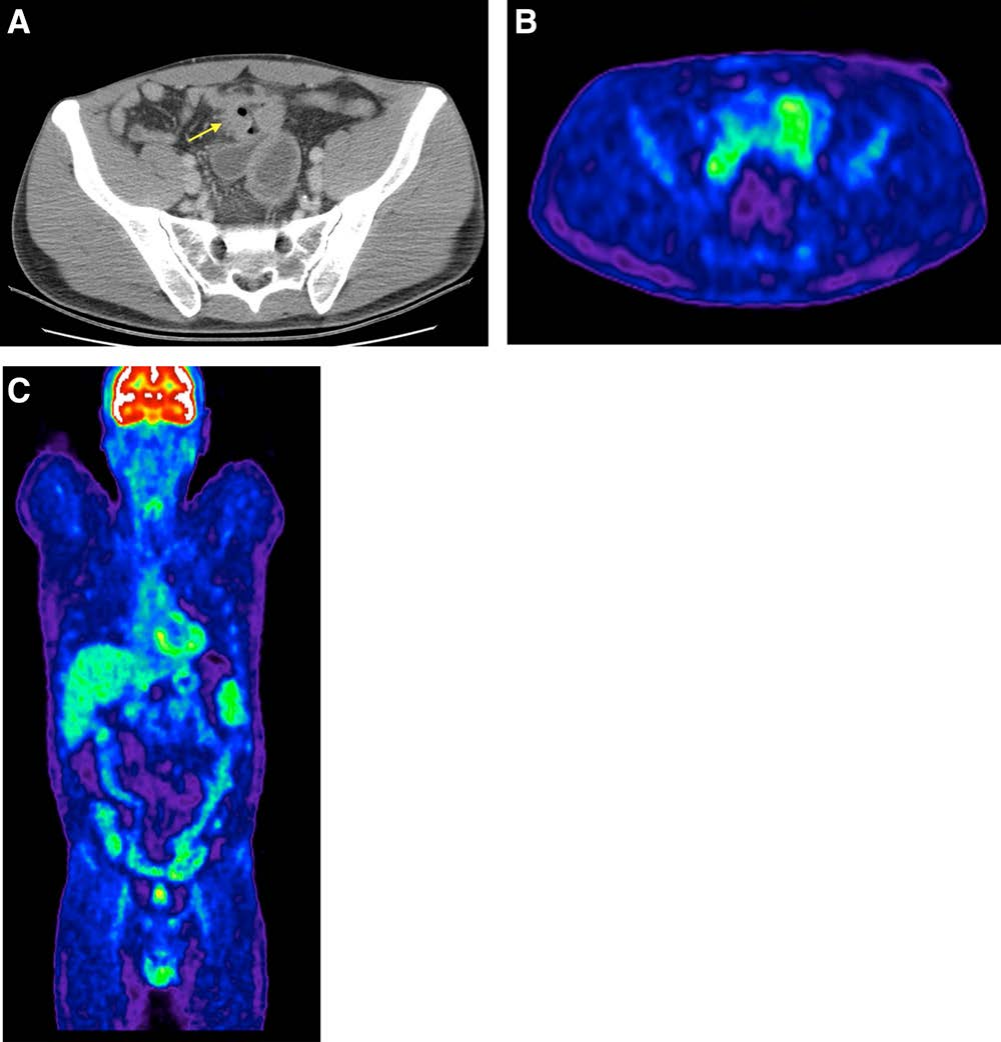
Abdominal CT scan and PET-CT. (A) Abdominal CT scan at the first diagnosis showed circumferential ileum wall thickening involving a short segment of a single bowel loop (arrow). (B), (C) Monitoring PET-CT after the haemato-oncological diagnosis: demonstrates increased metabolic activity at the level of a pelvic ileal loop. CT = computed tomography.
